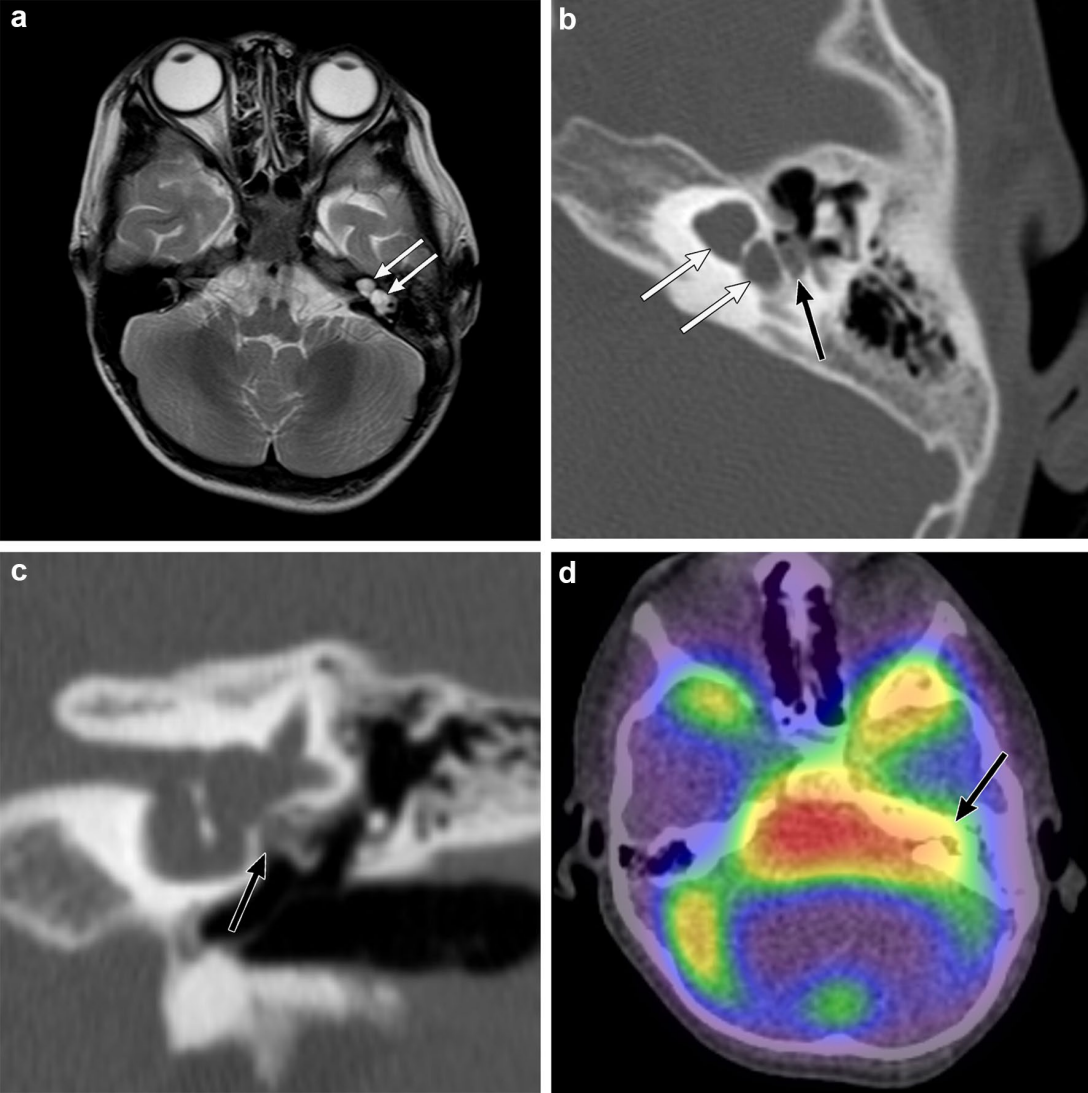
Left inner ear malformation (incomplete partition type I) in a 5-year-old boy with recurrent bacterial meningitis. The patient has left sensorineural hearing loss. a T2-weighted magnetic resonance image shows that the enlarged cochlea and vestibule in the left temporal bone suggest an inner ear malformation (white arrows). b Axial computed tomography (CT) of the temporal bone shows that the left cochlea has a cystic appearance and the vestibule is enlarged and cystic (white arrows). The cochlea and vestibule are separated, suggestive of incomplete partition type I. Fluid accumulation is observed in the lateral side of the left oval window, highly suggestive of a cerebrospinal fluid (CSF) leak (black arrow). c Coronal CT of the temporal bone shows fluid accumulation protruding through the left oval window (black arrow), suggestive of a CSF leak through a fistula in the left oval window associated with inner ear malformations. d Radionuclide cisternography with a Single-photon emission CT/CT fusion image shows increased radiotracer accumulation in the left temporal bone (black arrow), and a CSF leak into the left middle ear is confirmed. Leak of CSF from a partial defect of the stapes footplate is confirmed during the surgical repair
